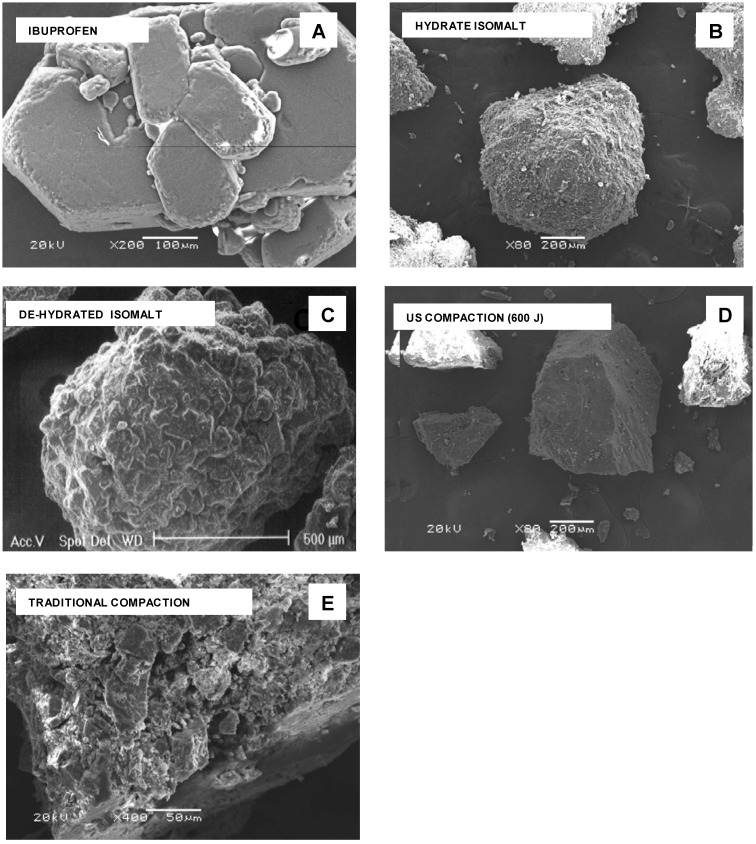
SEM photos. A – ibuprofen; B - hydrate isomalt; C – de-hydrated isomalt; D – tablet fragment from ultrasound-assisted compaction; E - tablet fragments from traditional compaction.
